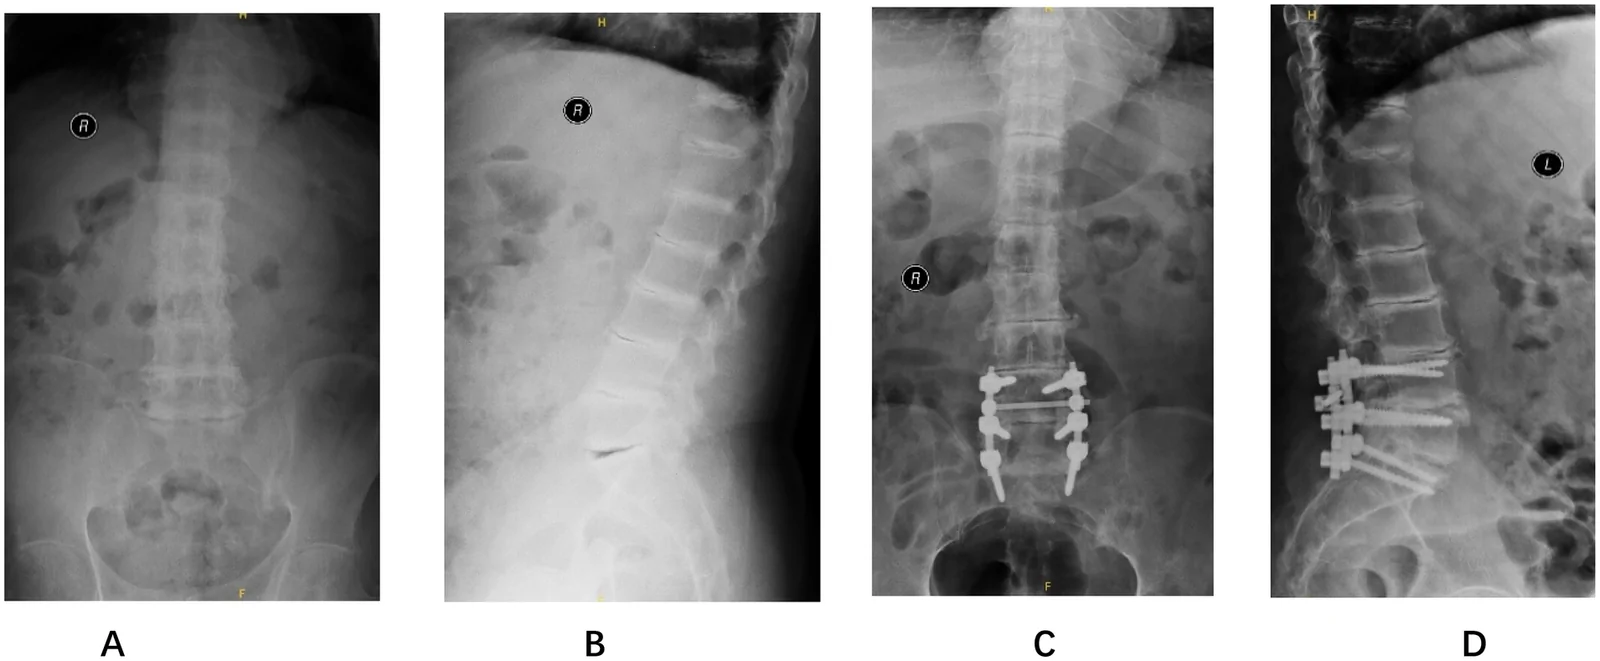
(A,B) Preoperative x-ray revealed loss of lumbar physiological lordosis with concomitant kyphosis, multilevel disc calcification, and vacuum phenomenon. (C,D) Postoperative anteroposterior and lateral x-ray.
